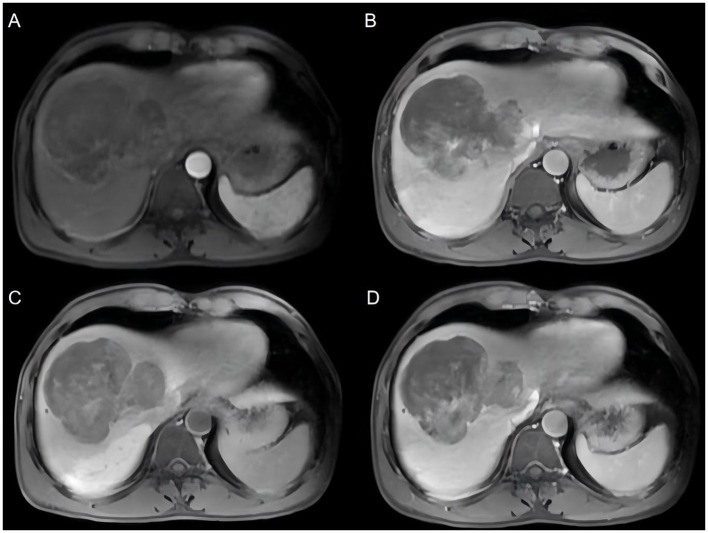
Magnetic resonance imaging (MRI) findings of the hepatic lesion. (A) Axial T1-weighted image demonstrates an iso- to slightly hypointense mass in the right hepatic lobe. (B) T2-weighted image shows a heterogeneous lesion with relatively hyperintense signal intensity. (C–D) Dynamic contrast-enhanced images reveal mild and heterogeneous enhancement of the tumor, with large non-enhancing areas consistent with extensive intratumoral necrosis. Overall, the lesion exhibits hypovascular imaging characteristics, which may mimic intrahepatic cholangiocarcinoma.
